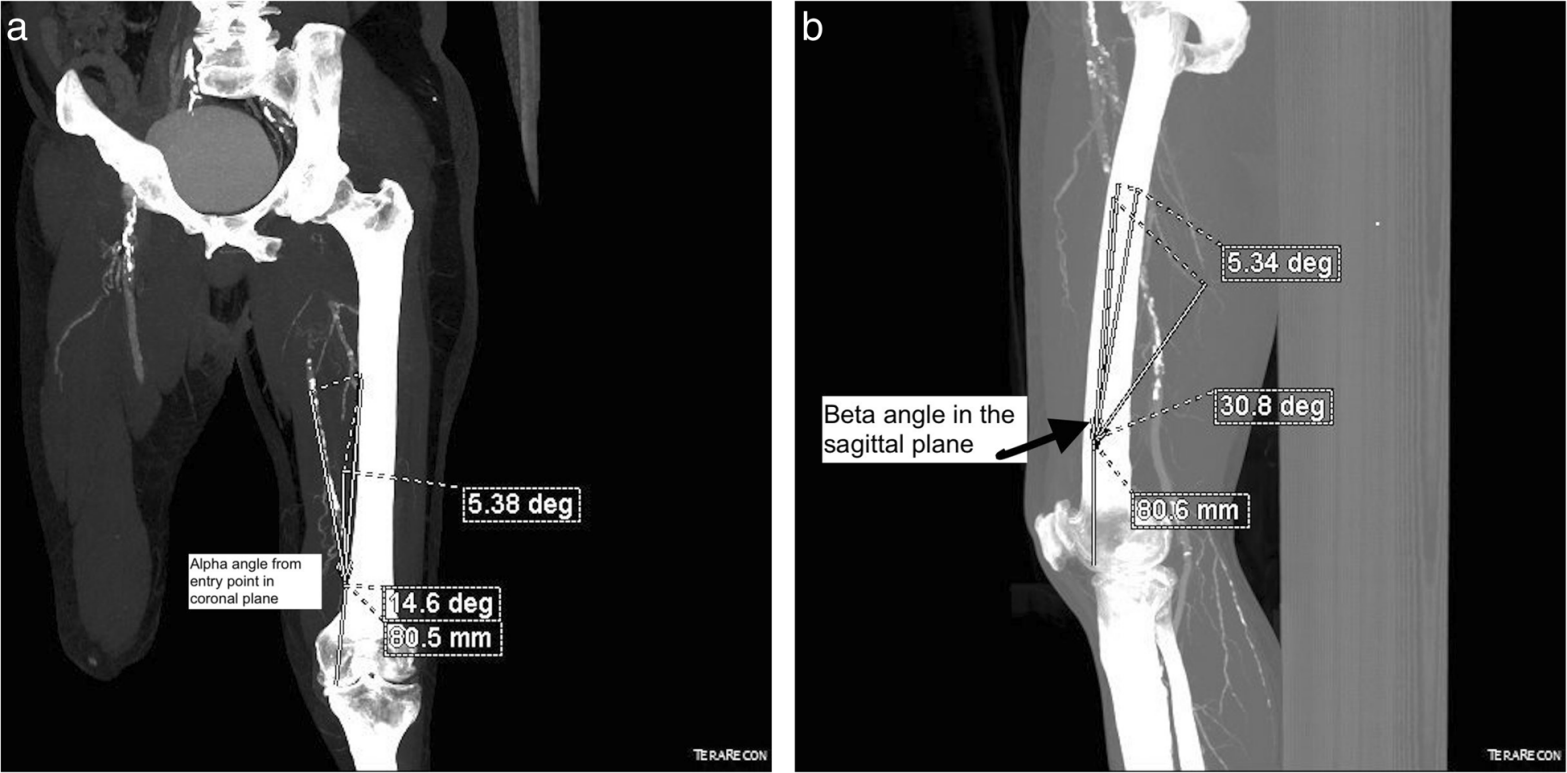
a Measurements of the alpha angle taken in computed tomography (CT)-angiogram reformatted images. In the coronal plane a line was drawn from the entry point (E) to the high medial point (M). The angle between this line and the line along the long axis of femur was termed the alpha 1 angle. Another line was drawn in the coronal section from the entry point (E) to the adductor hiatus (AH). The angle between this line and the line along the long axis of the femur was termed the alpha 2 angle. b Measurements of the beta angle taken in CT-angiogram reformatted images. Beta angle: in the sagittal plane a line was drawn from the E to the M. The angle between this line and the line along the long axis of the femur was termed the beta 1 angle. Another line was drawn from E to AH. The angle between this line and the long axis of the femur was termed the beta 2 angle
